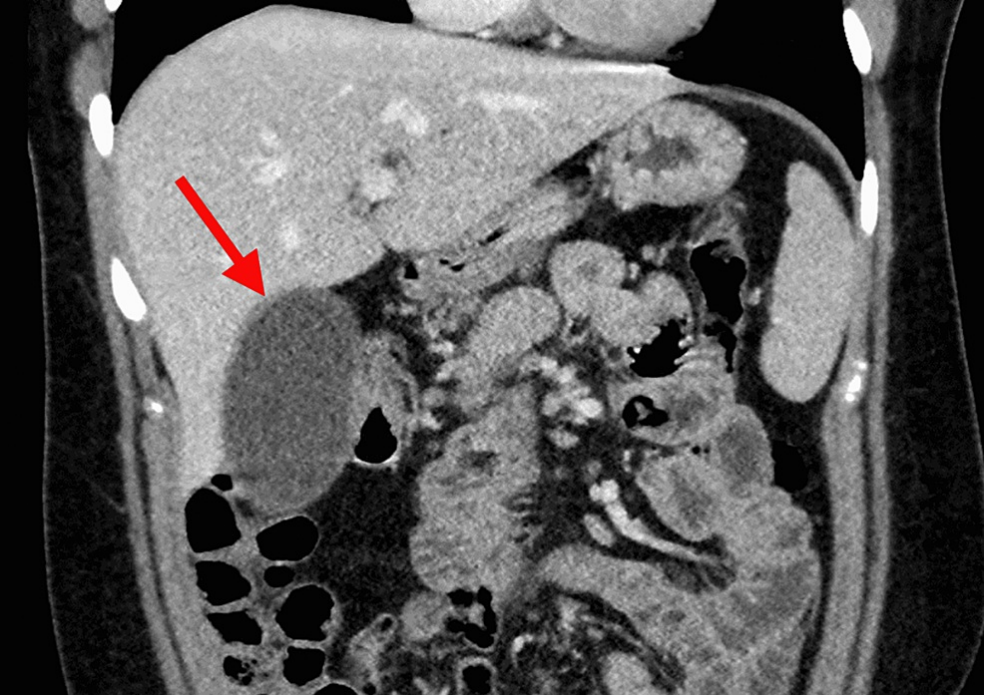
CT scan showing a distended and inflamed gallbladder. CT: computed tomography
